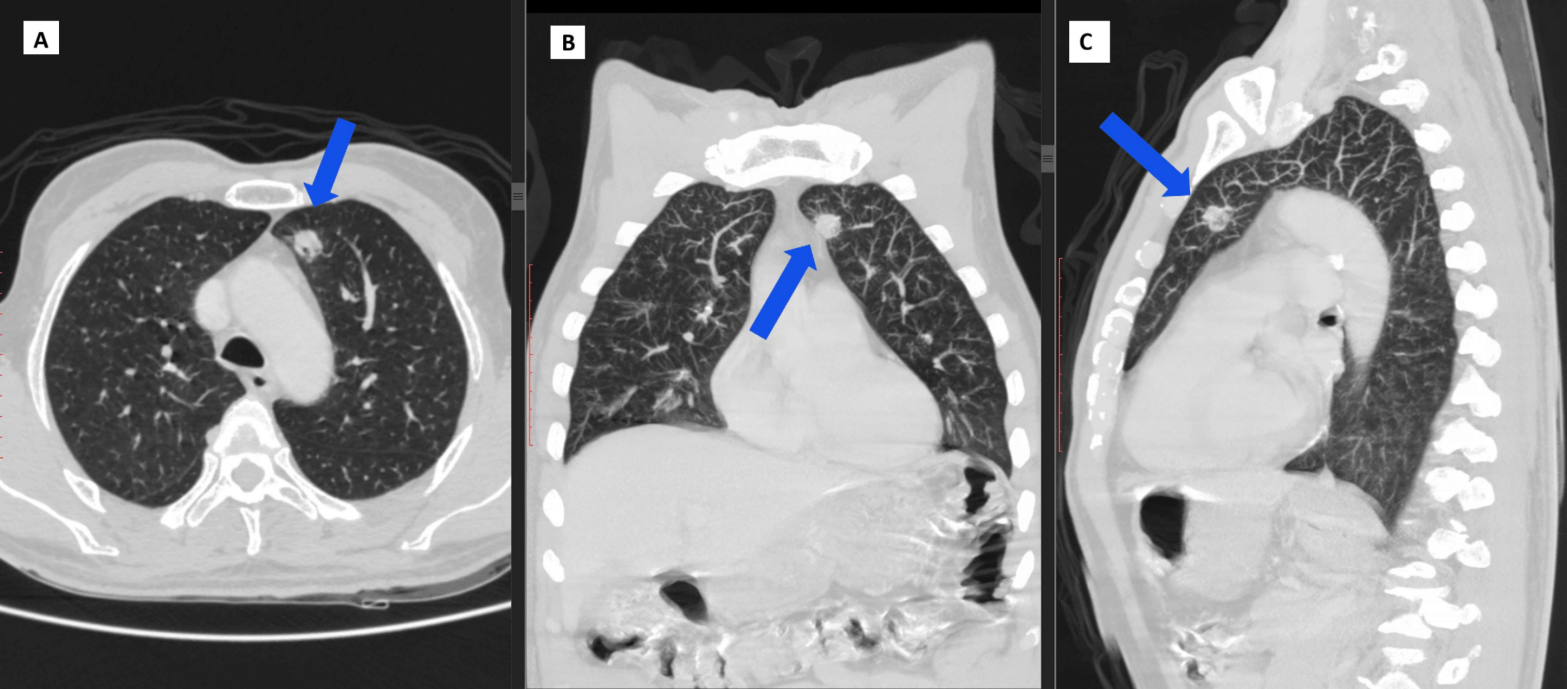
Contrast-enhanced CT chest showing a left upper lobe pulmonary mass (blue arrows) in axial (A), coronal (B), and sagittal (C) reconstructions. CT: computed tomography
